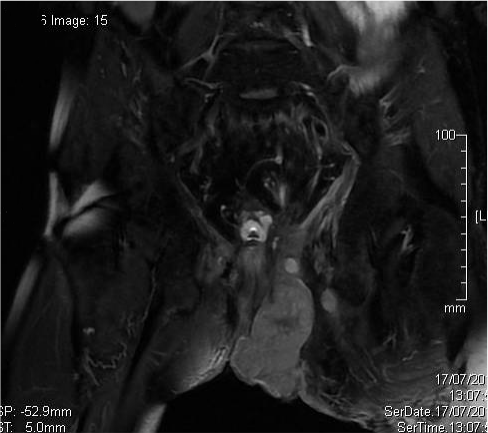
Dynamic postcontrast fat-suppressed coronal T1-weighted image: Lobulated left sided perineal mass measuring about 7 cm connected to the anal canal, with mild heterogeneous enhancement, it's associated with perineal fat infiltration and left side gluteal abcess
